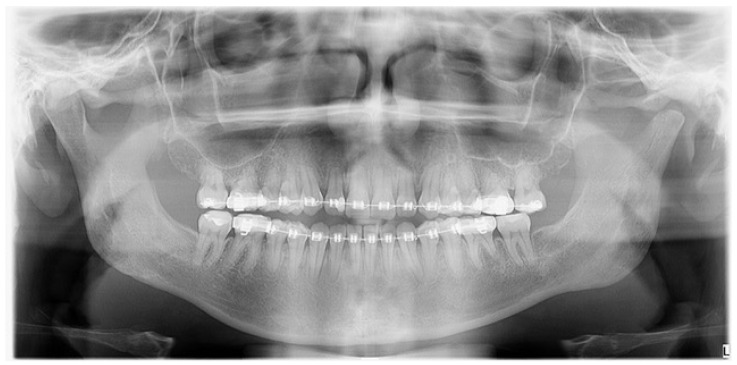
A control panoramic radiograph after six months after a left-sided condylectomy. The jawbone remains asymmetrical with a deviated midline and inappropriate bite; therefore, the patient was scheduled for a revision BSSO surgery to establish more accurate jaw proportions. The scope and degree of the excised affected condylar head influences the later shape and size of the newly formed condylar head stump. Most authors recommend its 5 mm bone removal; however, in this presented case and in others, surgery excision must be bigger and is case-related. Achieving a stable position of the condylar head inside the glenoid fossa is an important matter [3,4,5].
